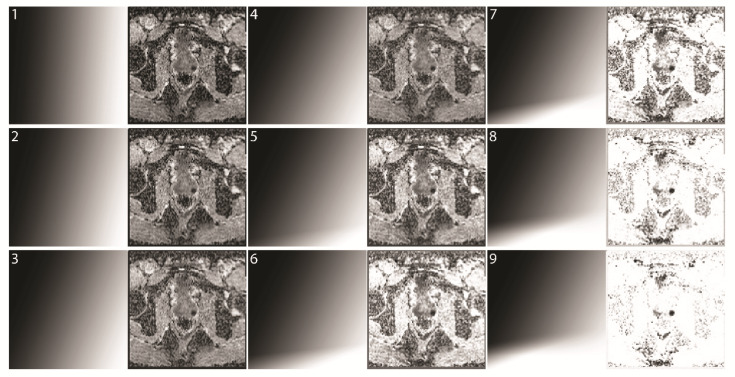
Increasing strengths of background suppression. Examples of different readouts from a mcDI dataset created from hbDWI and the corresponding ADC (map 1–9). The selected map is shown on the left and the resulting mcDI on the right. Map 1 has a strict vertical readout and therefore yields the original ADC image. In the following maps, the gradient is tilted clockwise in increments to include the DWI value. Additionally, another gradient is added at the bottom to suppress the background.
